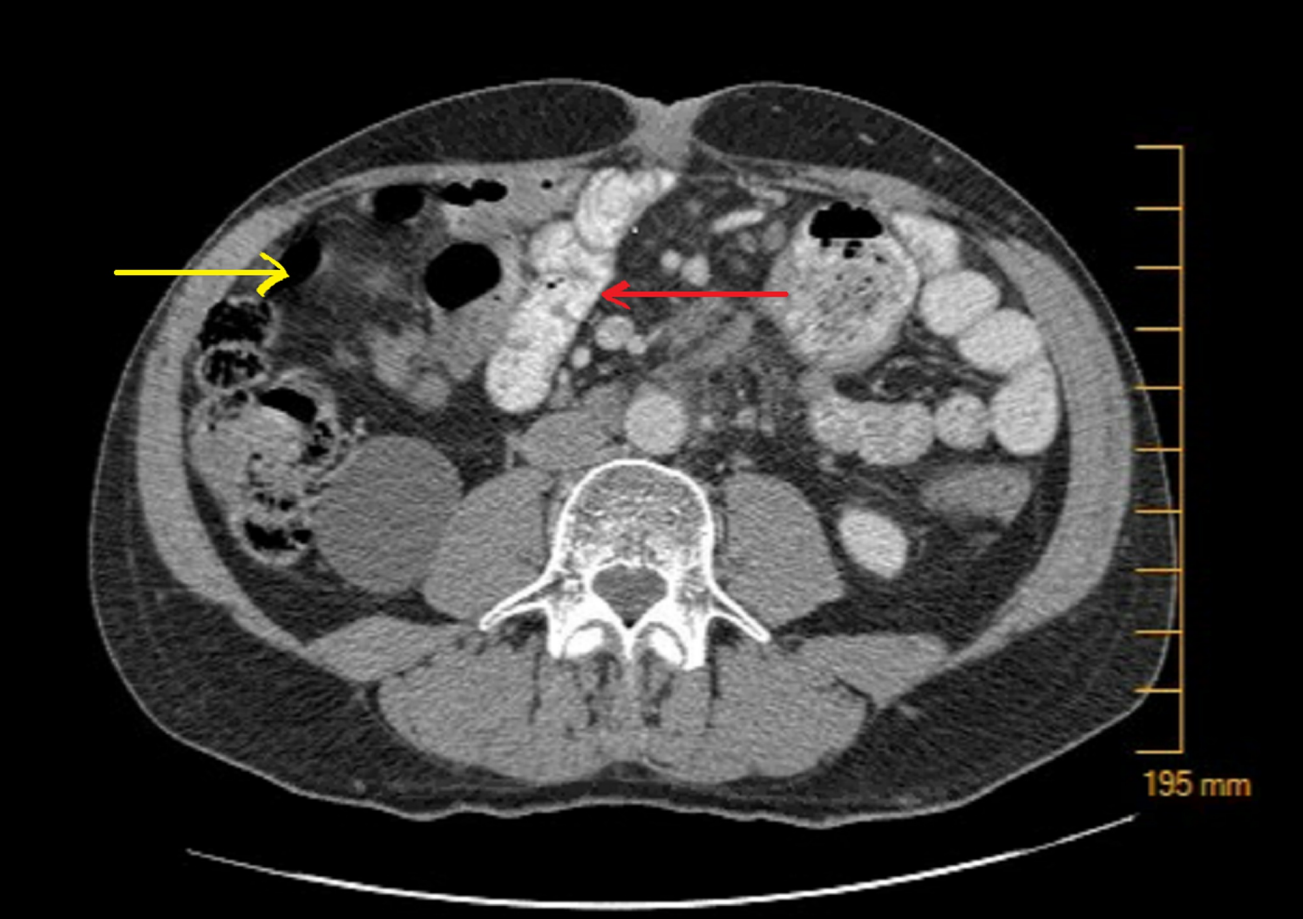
CT abdomen showed a 3.7 x 2.5 x 3.1 cm density at the root of mesentery anterior to the aorta at the level of the distal duodenum (Red Arrow), Dilated intrahepatic biliary ducts (Yellow arrow)
